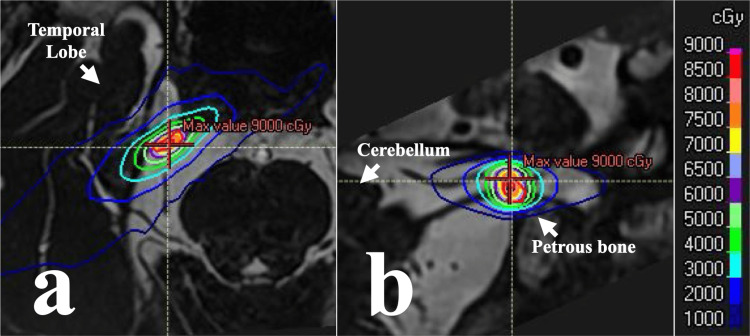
Dose distribution of SRS (Case 2). Isodose lines are shown relative to the maximum dose (100% = 90 Gy) on high-resolution T2WI. (a) Axial view and (b) sagittal view. SRS: stereotactic radiosurgery; T2WI: T2-weighted imaging.
